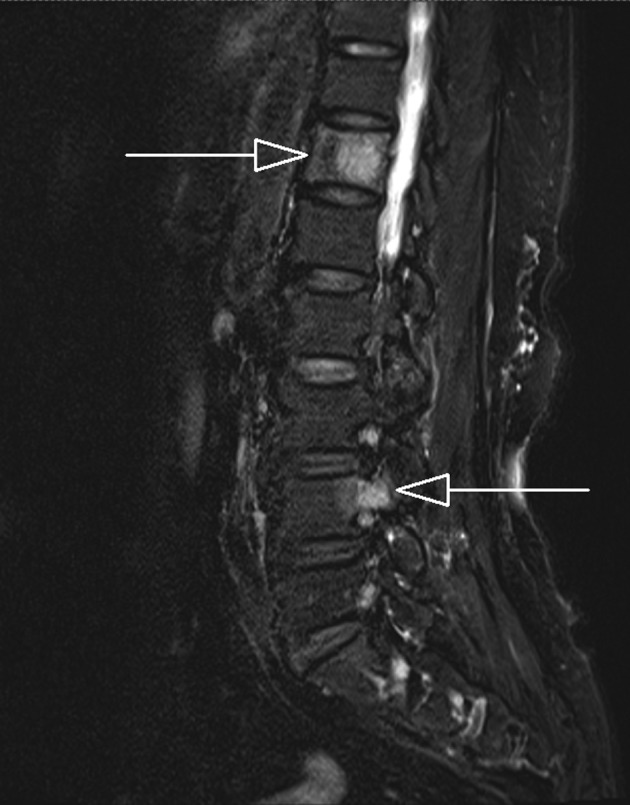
Magnetic resonance imaging showing the 12th thoracic vertebra and the pedicle of the 4th lumbar vertebral arch with an abnormal signal.
